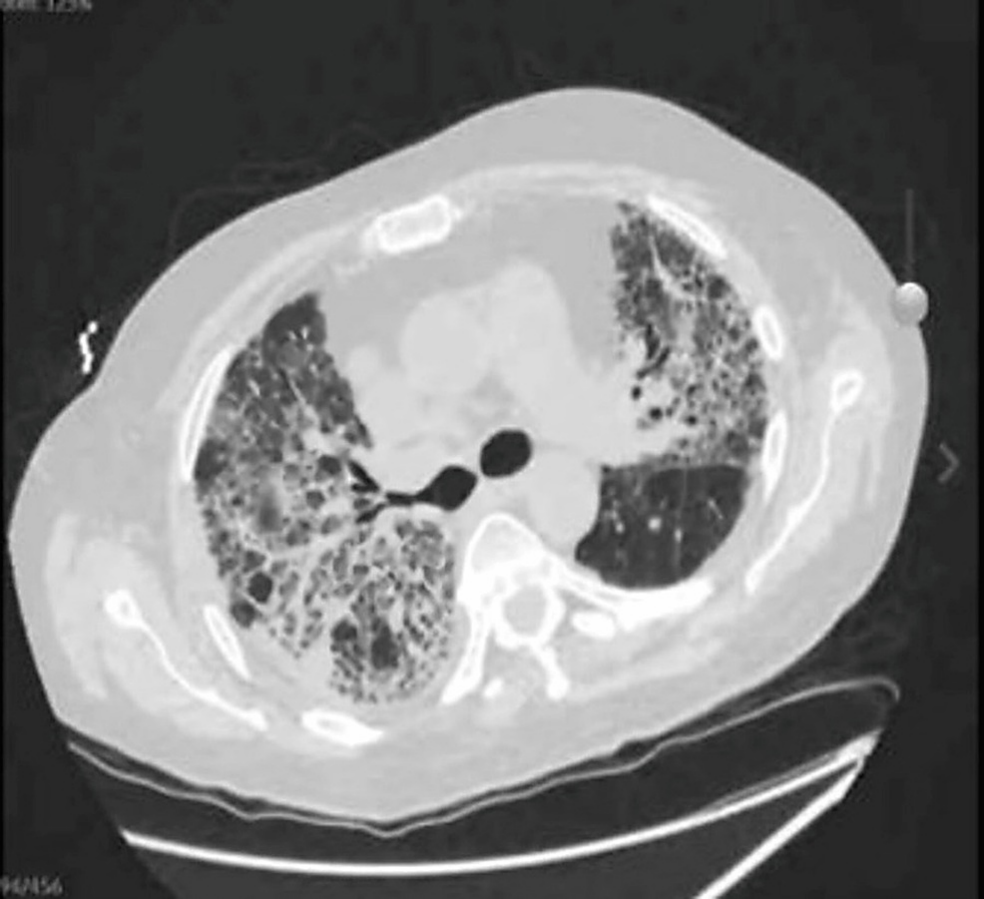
Axial view of CT chest demonstrating severe multifocal pneumonia with interval development of bronchiectasis
